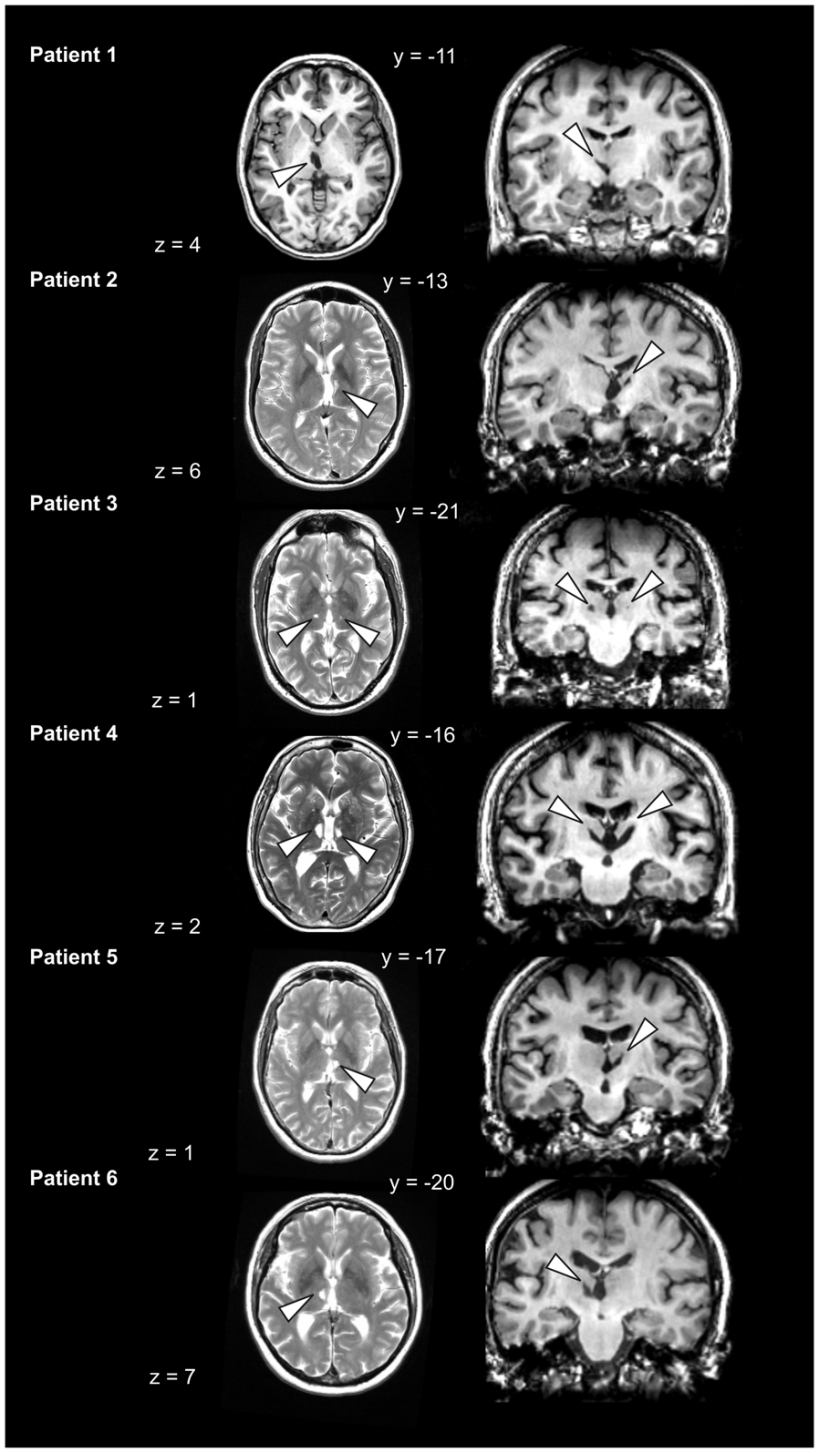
Transverse and coronal structural MR-images and lesion locations for all patients. T2-weighted transverse and T-1 weighted coronal MR images of lesion locations in individual patients (lesion locations are marked with white arrows). Note that for Patient 1 the transverse slice is also provided T1-weighted. MRI showed left-hemispheric damage to the thalamic region in Patient 1 and Patient 6, right-sided lesions in Patient 2 and 5 and bilateral damage in Patient 3 and Patient 4.
